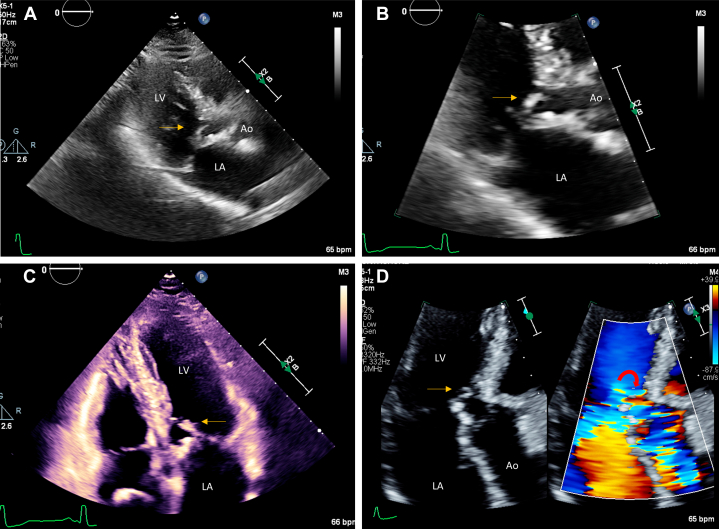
Findings on Initial Transthoracic Echocardiography (A) Parasternal long-axis view and (B) zoomed-in parasternal long-axis view with calcified anterior mitral valve leaflet with systolic anterior motion (yellow arrow). (C) Apical 5-chamber view with systolic anterior motion (yellow arrow) and (D) Apical 3-chamber view with systolic anterior motion (yellow arrow) and resultant posteriorly directed mitral regurgitation and flow acceleration through the LVOT on color Doppler (red curved arrow). Ao = aorta; LA = left atrium; LV = left ventricle; LVOT = left ventricular outflow tract.
